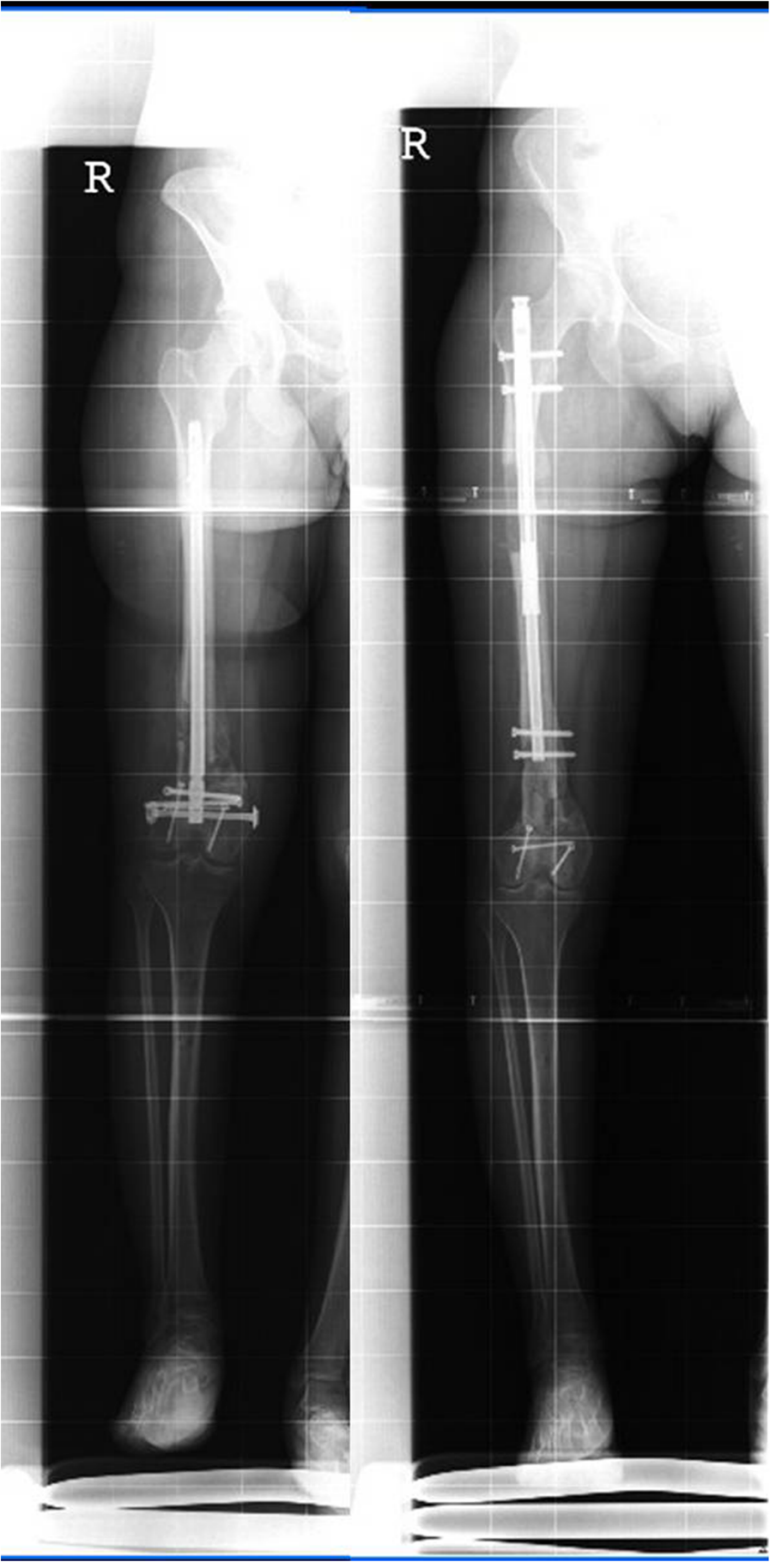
Full leg X-rays before and after distraction.
